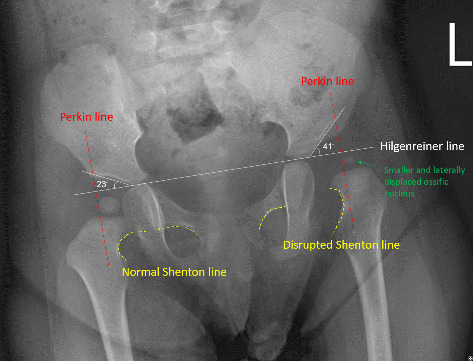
The X-ray taken at 11 months revealed disrupted Shenton line, increased acetabular index, subluxation of the left hip, and a small ossific nucleus of the capital femoral epiphysis in the left hip.
